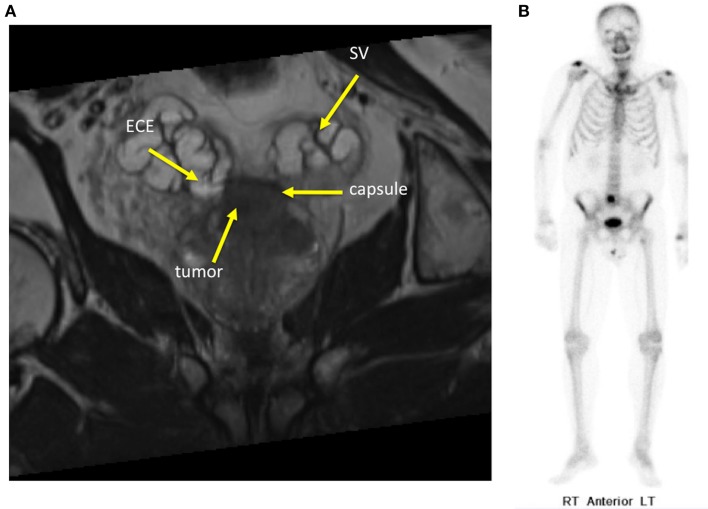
Sixty-year-old gentleman with oligometastatic prostate cancer. He presented with back pain and his PSA was 35 ng/ml. DRE was abnormal and imaging revealed: (A) Coronal T2-weighted multiplanar reconstruction MRI image demonstrating extracapsular extension into the seminal vesicles. (B) Bone scan demonstrating a solitary L5 vertebral body metastasis.
